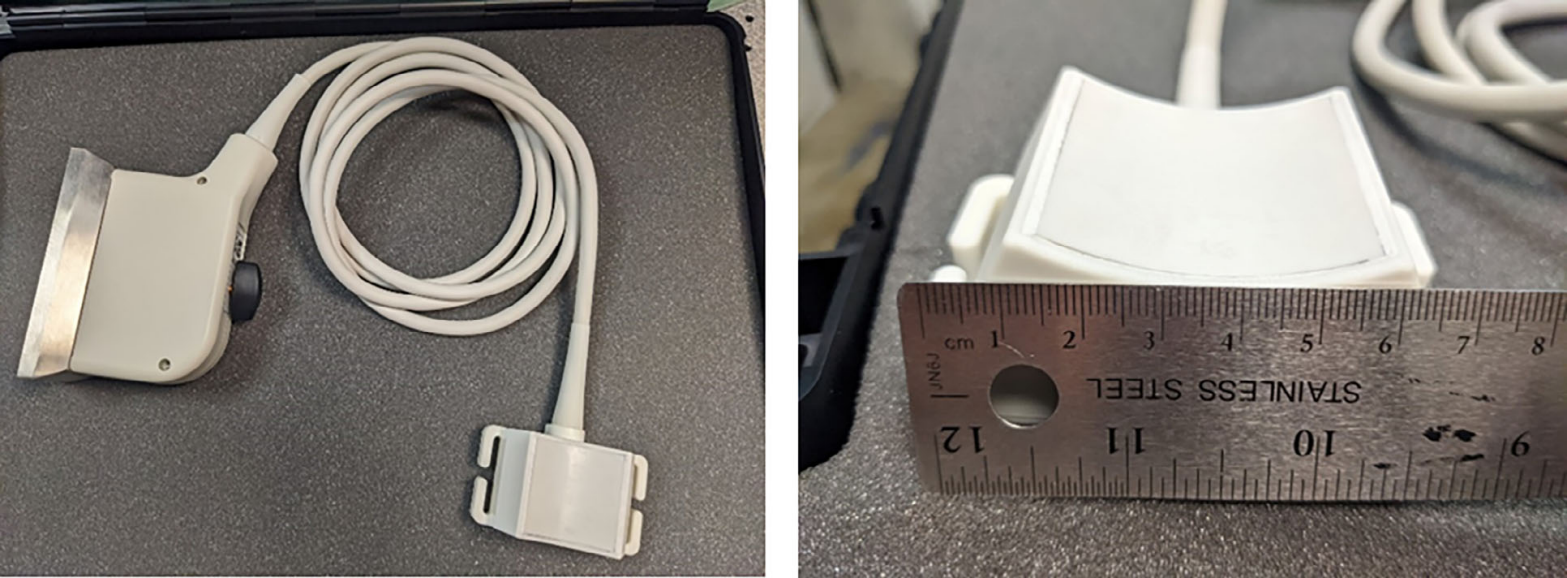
Custom curved‐matrix transcranial ultrasound array.
